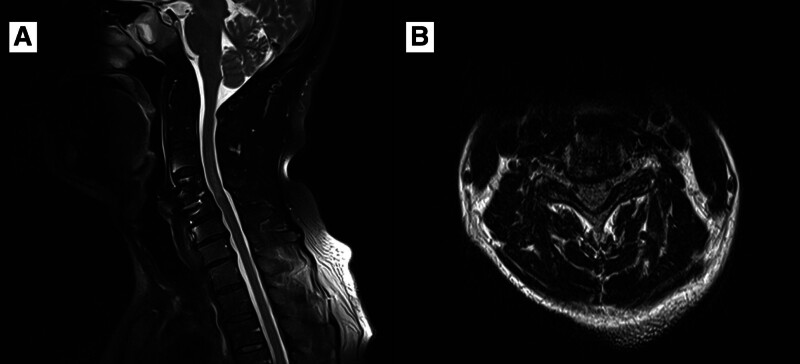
Postoperative magnetic resonance imaging in sagittal T2-weighted (A) and axial T2-weighted (B) sequences demonstrates C4-C5 anterior cervical discectomy and fusion without cord compression.
